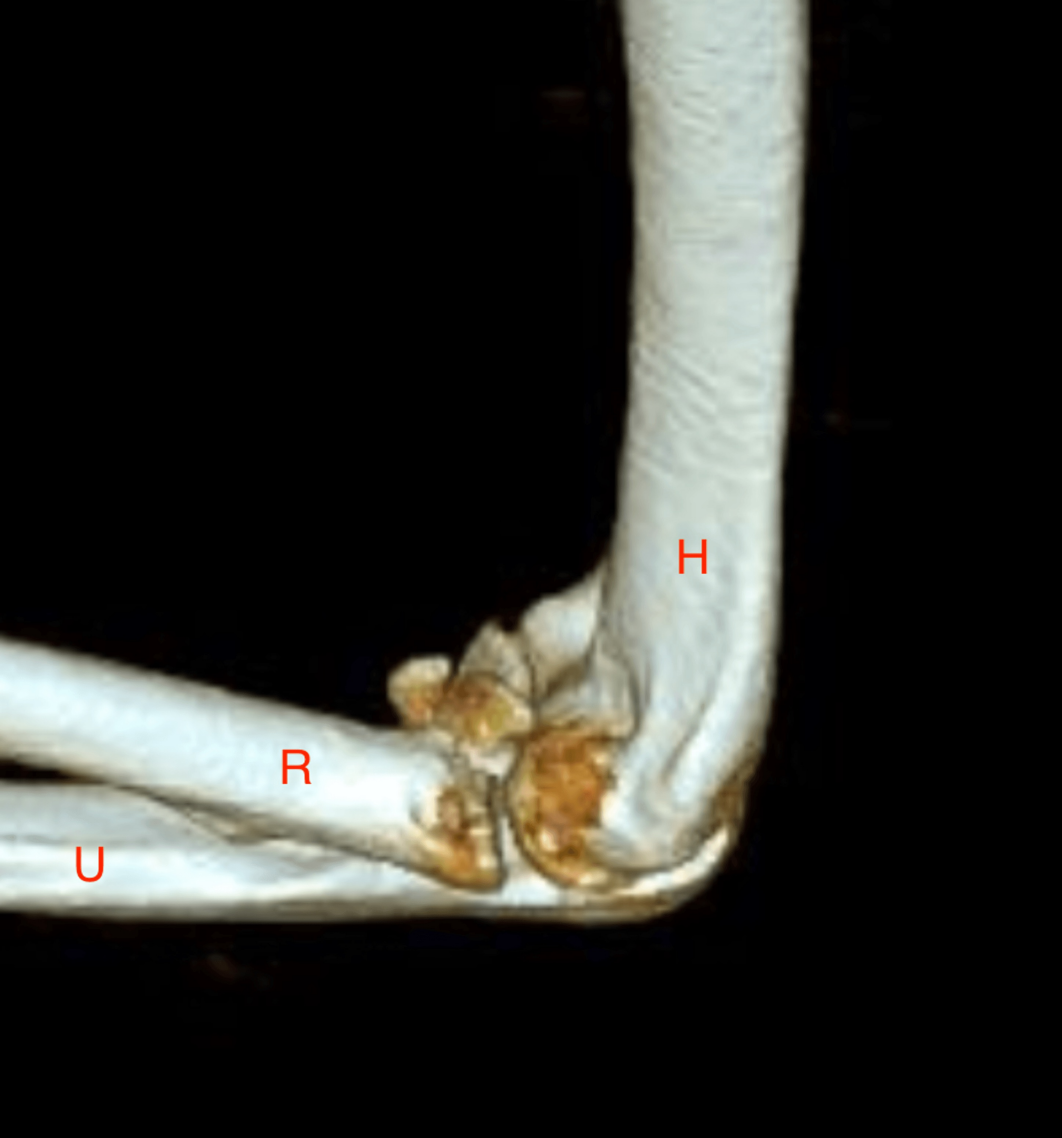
Computed tomography (CT) of the left elbow showing a comminuted fracture (highlighted in yellow). H: humerus; R: radius; U: ulna
